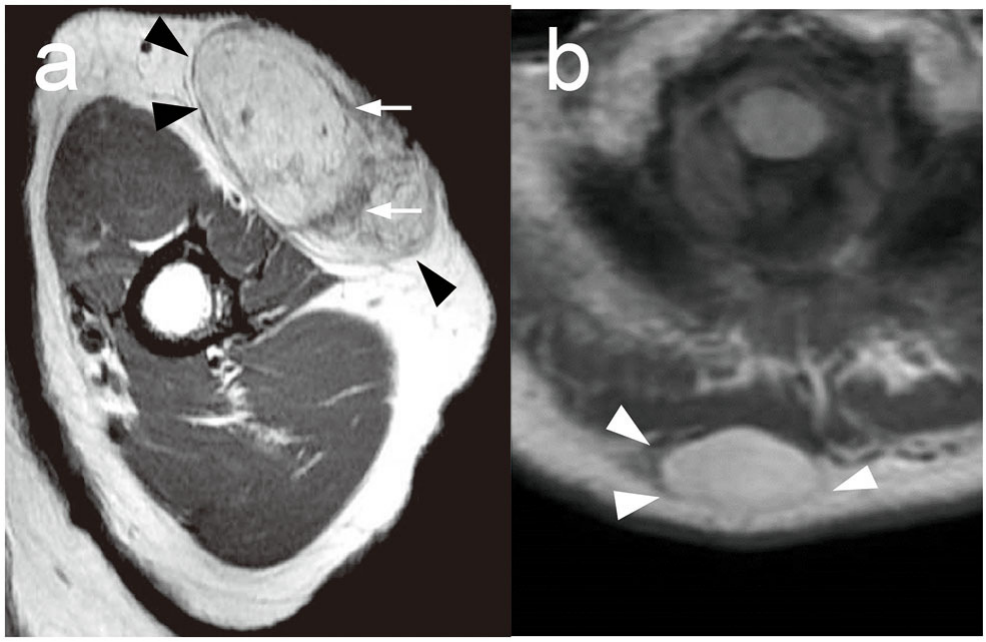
Representative MRI findings in ALT and lipoma. (a) A representative MRI of superficial type ALT in the left arm. T1-weighted MRI showed a hyperintense subcutaneous mass with a well-defined smooth margin (arrowheads). Thick hypointense septa (arrows) were recognized. (b) A representative MRI of lipoma in the back. T1-weighted MRI showed a hyperintense subcutaneous mass with a well-defined smooth margin (arrowheads) without hypointense septa.
